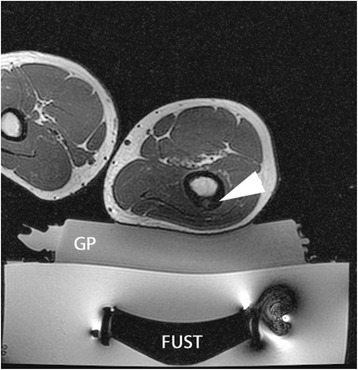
Focused ultrasound therapy setup. Axial T1-w MRI showing osteoid osteoma of right lateral femoral diaphysis (*white arrowhead*) placed onto gel pad (*GP*) overlying ultrasound transducer (*FUST*)
